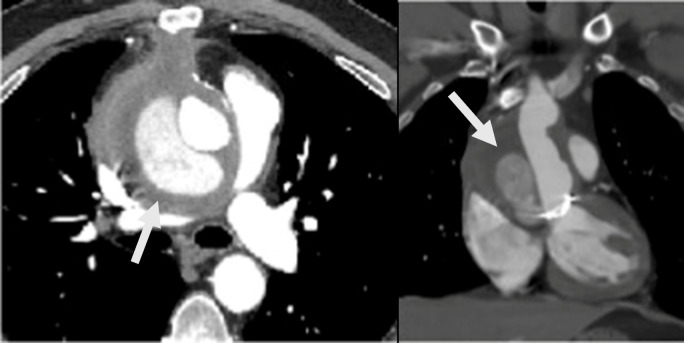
Pseudoaneurysm CTA. Initial chest tomography angiography axial (left) and coronal (right) views. There is a dilated ascending thoracic aorta and aortic root, measuring up to 7.6 × 7.2 cm transaxially, with aortic dissection extending from the aortic root to the mid-t-distal ascending thoracic aorta, with associated opacification of the false lumen with contrast. Additional intramural and extramural hematomas are visualized, concerning for pseudoaneurysm vs rupture vs impending rupture, requiring further evaluation by cardiothoracic surgery (arrows).
